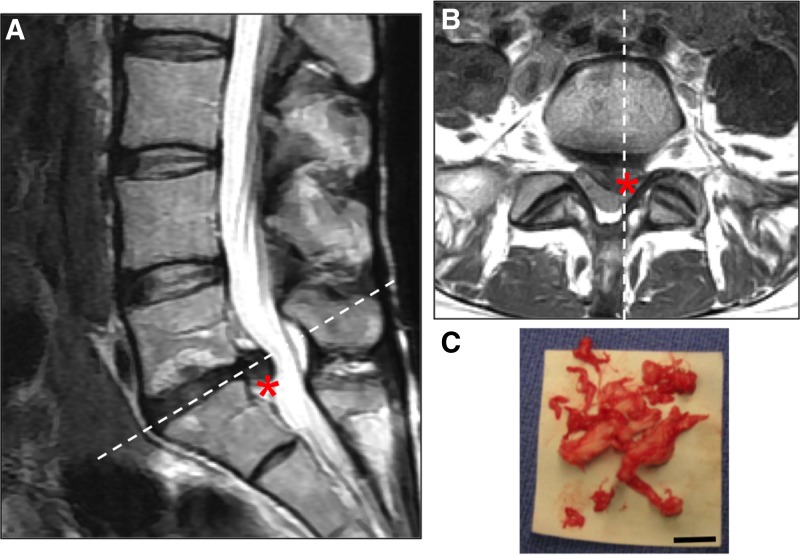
(A) Midsagittal and (B) axial sections of T2-weighted MRI from a 42-year-old female with lumbar radiculopathy. The white dashed lines indicate the plane of orientation in the axial and midsagittal views, respectively. The patient had a left-sided paracentral disc herniation with compression of the traversing S1 nerve root (represented by *). (C) Photograph of disc material successfully removed during a surgical procedure to treat painful disc herniation. Scale bar represents 20 mm.
